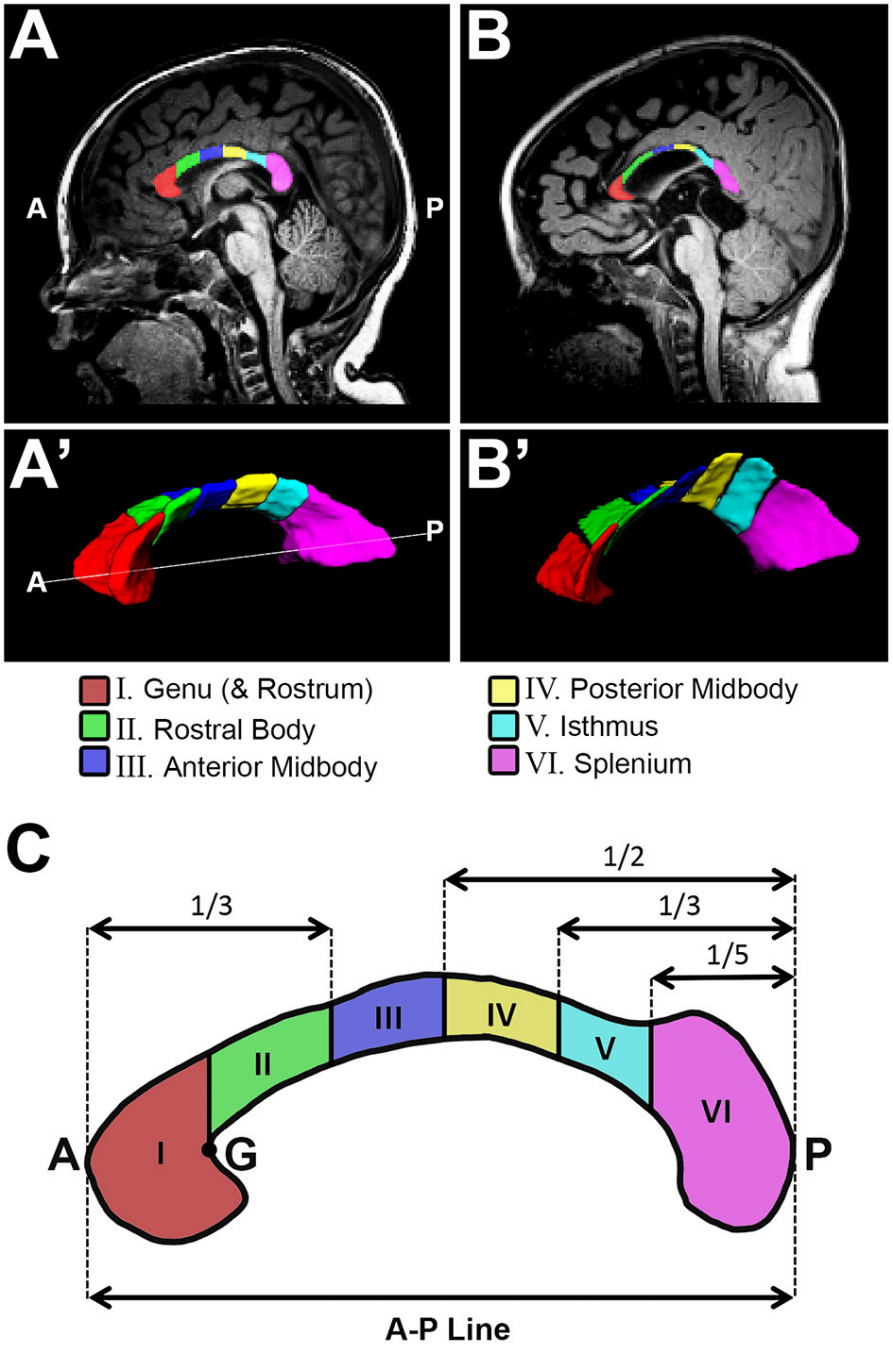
Sub-regional corpus callosum analysis. Representative T1-weighted corpus callosum (CC) segmentation for a term-born otherwise healthy control (A,A') and a term-born patient (B,B') scanned at 10 and 9 months of age, respectively. Volumes of CC were estimated for a total of 6 CC sub-regions (C). Sub-regional segmentation used the proportion-based parcellation scheme originally proposed by Witelson (23), with slight modification based on Venkatasubramanian et al. (37) (see Methods for details). A, anterior; G, genu; P, posterior.
